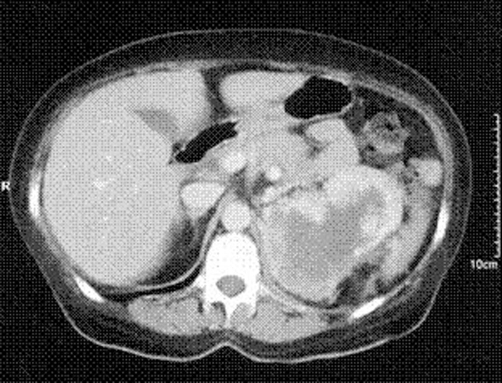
Contrast CT scan of the Left Renal Cell Carcinoma revealing a 10 × 8 cm intensely enhancing heterogeneous mass arising from the upper pole.
